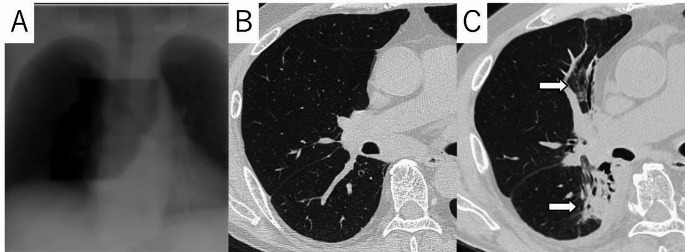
Radiation Recall Pneumonitis in a 71 years-old woman with lung cancer. Portal radiograph shows an irradiated field (A). High-resolution CT (HRCT) image taken one month after irradiation showed no shadows corresponding to the irradiated field. However, HRCT image taken after starting immune checkpoint inhibitor (duruvalmab) showed ground-glass opacities and consolidations (arrows) corresponding to the irradiated field. RRP was diagnosed
